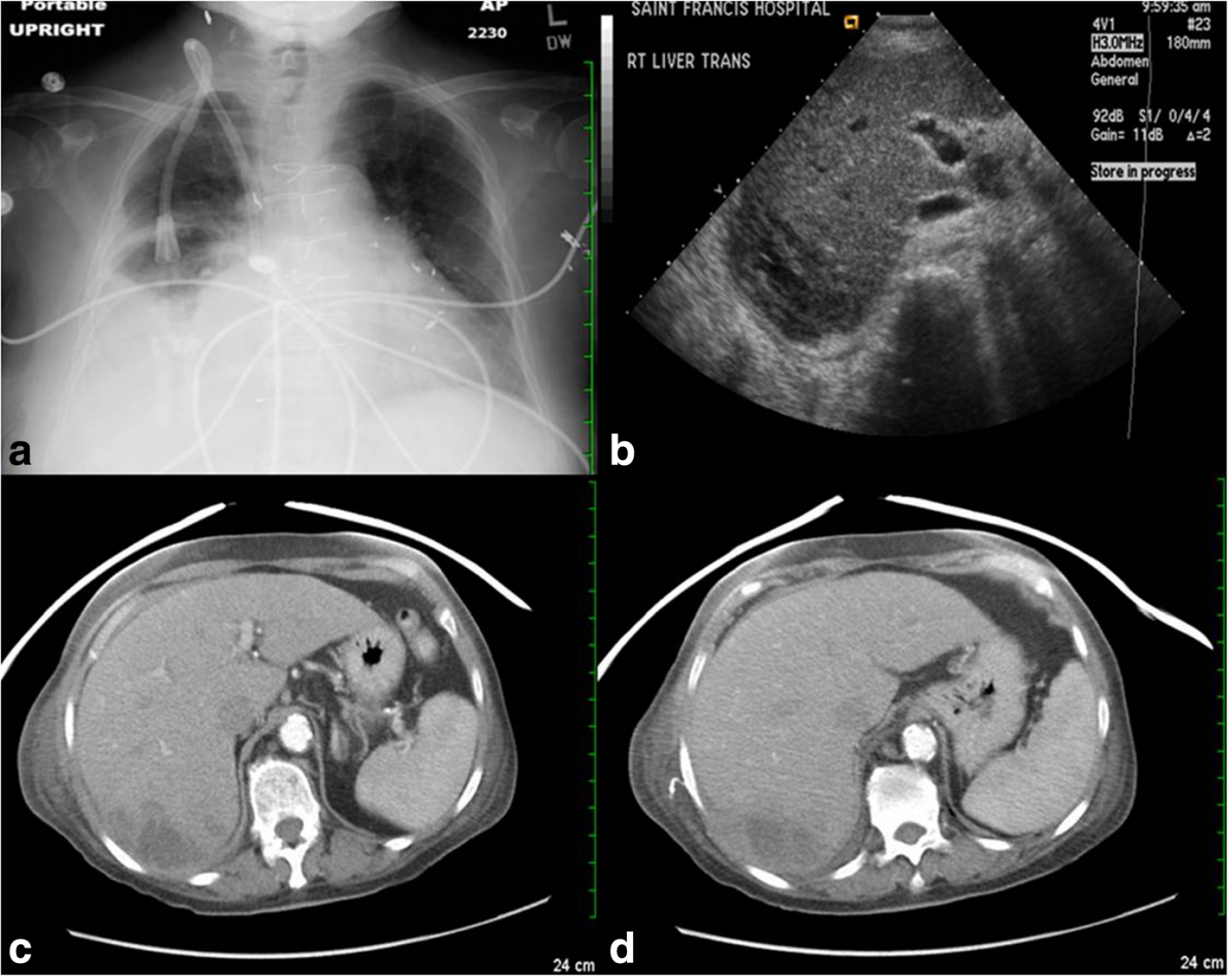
a CXR shows elevated right hemi-diaphragm with right lower lobe infiltration and pleural effusion. b U/S of RUQ shows hypodensity multiloculated mass in posterior aspect of right hepatic lobe. c, d CT scan of abdomen shows 6.6 × 4.7 cm hypodensity multiloculated mass in posterior aspect of right hepatic lobe
